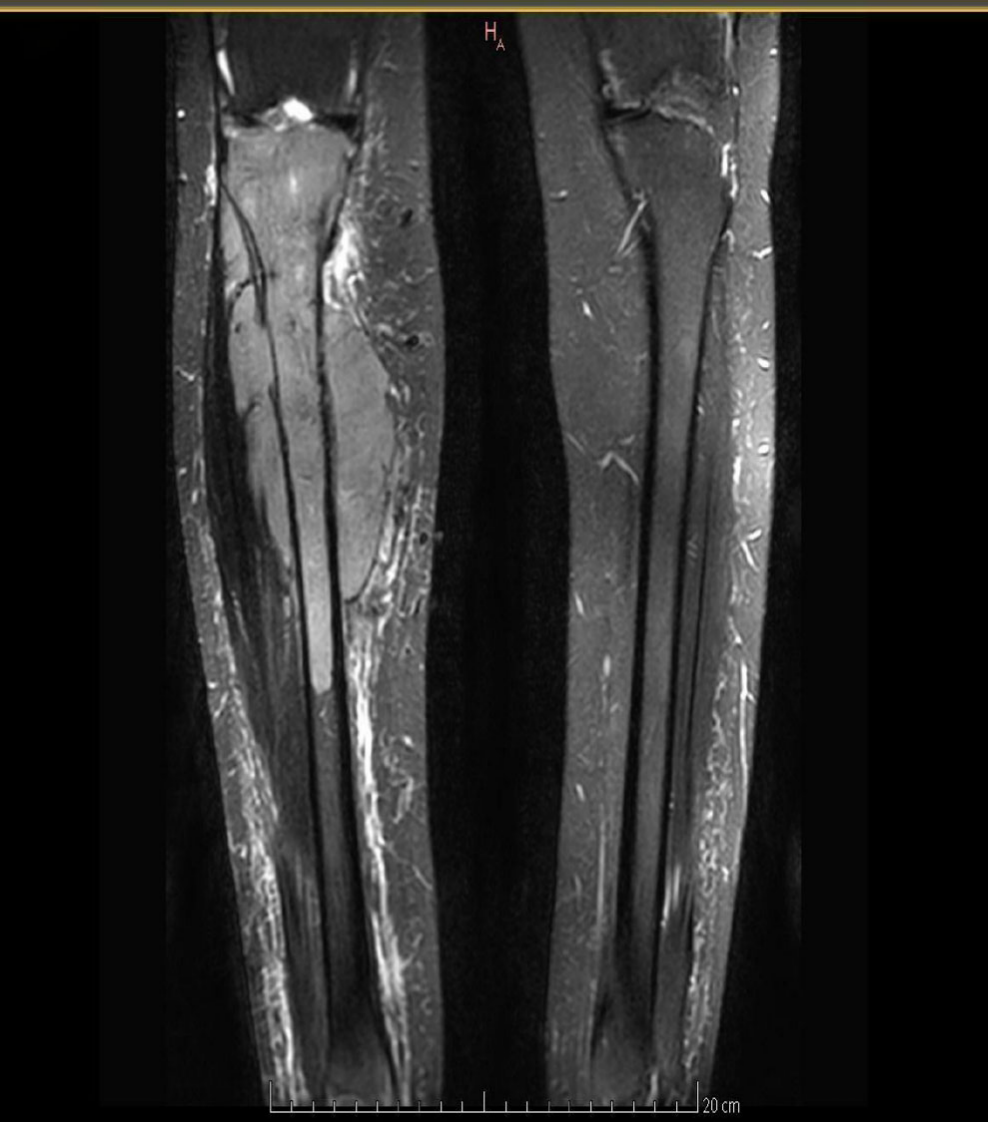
MRI of the legs showing a well-defined lobulated lesion on the right tibia (arrow), suspicious for plasmacytoma.
